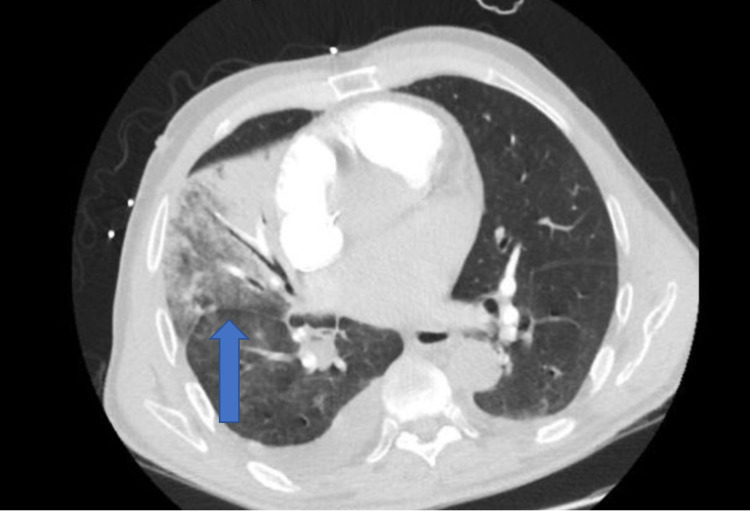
CTA of the Chest Revealing Right Pulmonary Infiltration in the Right/Middle Lobe With Basilar Parapneumonic Effusion (Blue Arrow). CTA: computed tomography angiography
